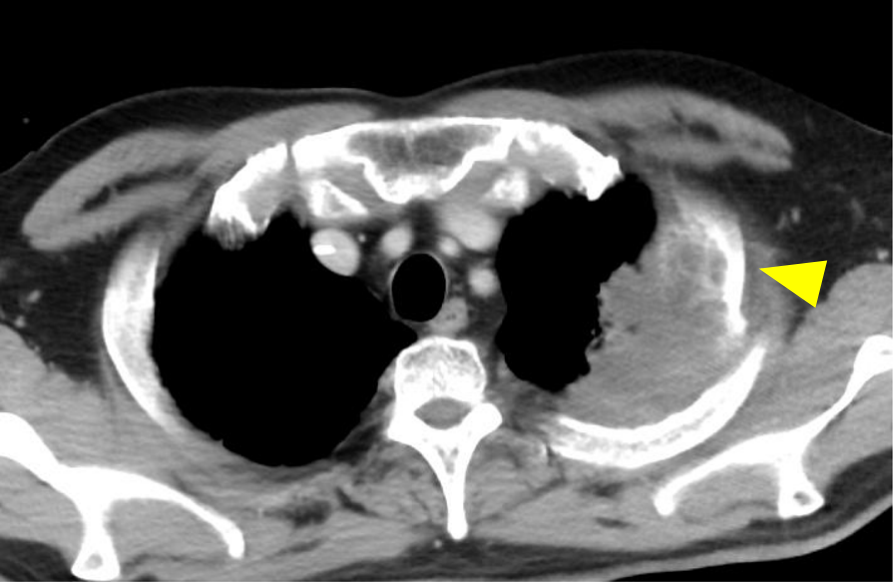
Thoracic CT scan. CT revealing an 80 × 60 mm mass lesion at the left upper lobe, which invaded the second rib (arrowhead)
